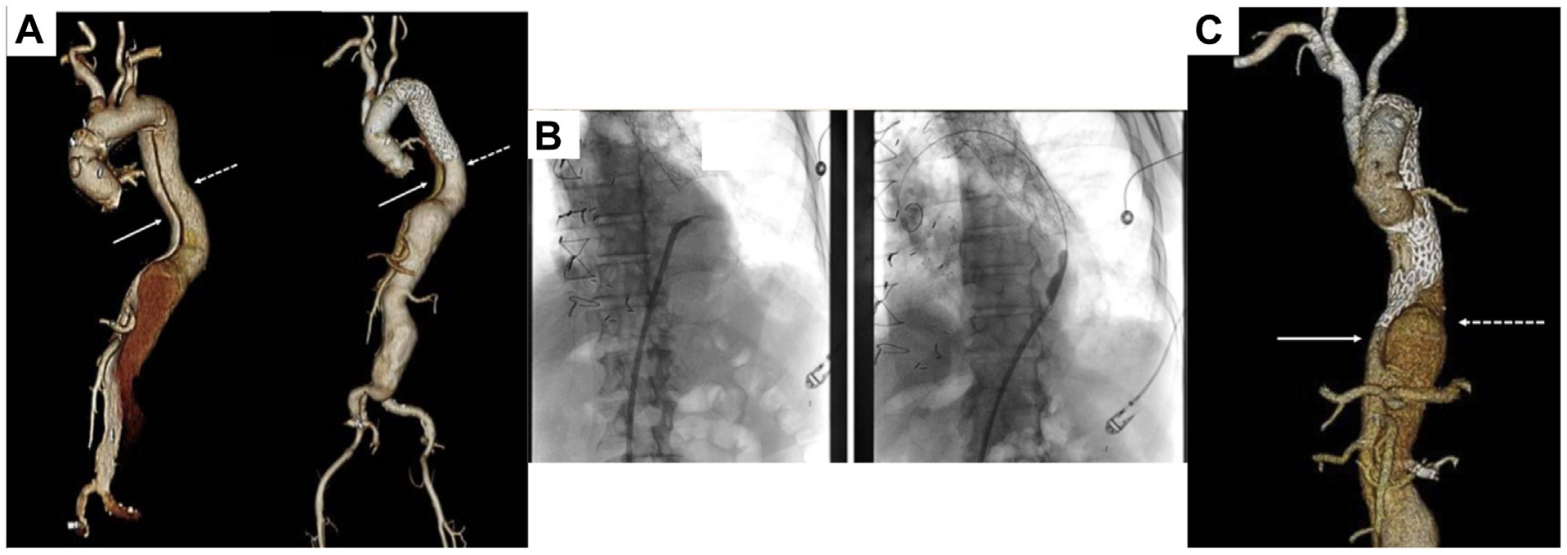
(A) Reconstructed three-dimensional computed tomography angiography (CTA) scan showing the pre- and postoperative images of patient with residual descending thoracic aortic dissection with subsequent false lumen deployment of frozen elephant trunk. The *solid arrows* point to the true lumen, whereas the *dashed arrows* point to the false lumen. (B) PowerWire (Baylis Medical) piercing through septum, cannulation of prior thoracic endovascular aortic repair endograft (TEVAR), and ballooning of septal fenestration. (C) Postoperative reconstructed three-dimensional CTA showing appropriate apposition of thoracic endovascular aortic repair (TEVAR) extending into the true lumen. (Reproduced from Plotkin A, Hanks SE, Han SM, Fleischman F, Weaver FA, Magee GA. Endovascular septal fenestration using a radiofrequency wire to salvage inadvertent false lumen deployment of a frozen elephant trunk stent graft. J Vasc Surg Cases Innov Tech 2019;5:553-556).
